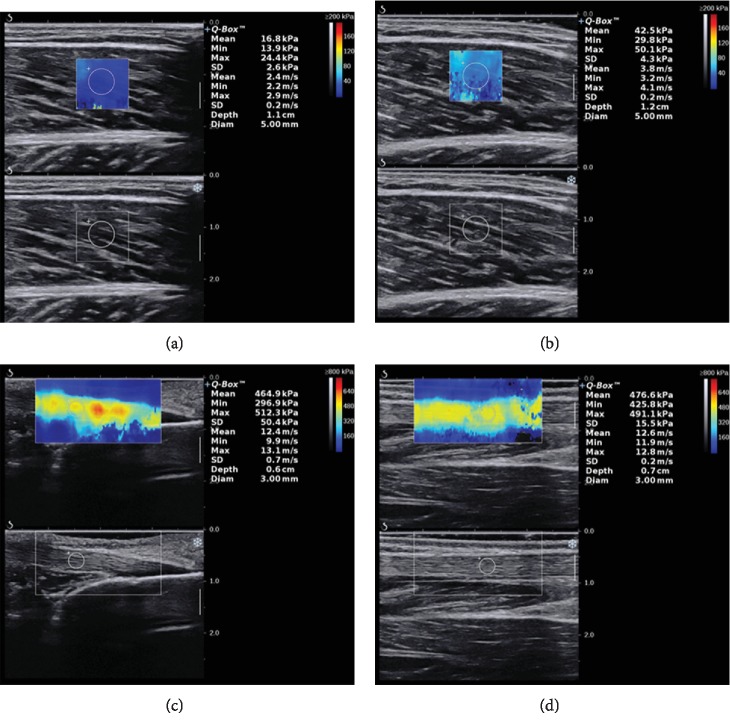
Changes of the elastic modulus of MG converted from the resting ankle angle to the neutral anatomical position (from (a) to (b)). The elastic modulus of AT0cm and AT3cm in the resting ankle angle (from (c) to (d)). The color-coded box presentation of muscle-tendon elasticity is shown in the upper images. The longitudinal grey scale sonograms of the muscle tendon are shown in the bottom images. The Q-Box™ is shown on the right.
